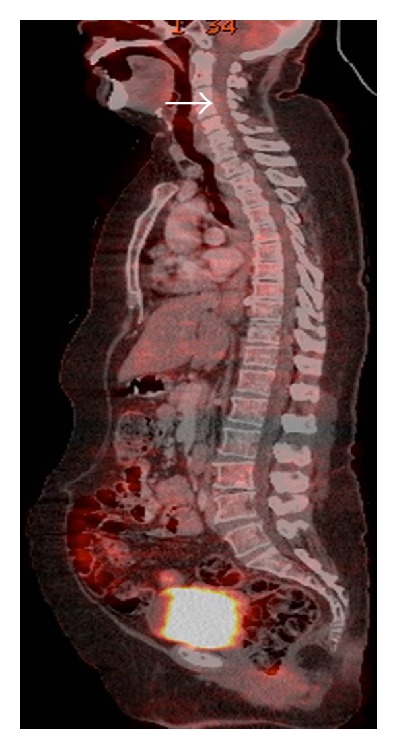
PET image showing moderately FDG avid intradural mass lesion (arrow).
